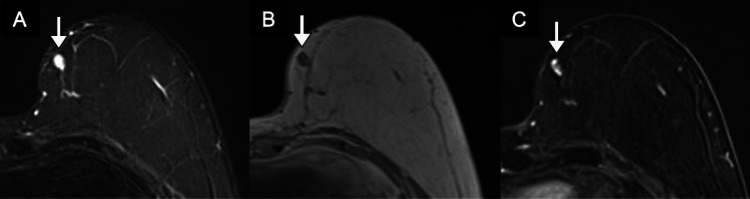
Surveillance magnetic resonance imaging of the left breast. (A) Axial STIR, (B) pre-contrast T1, and (C) post-contrast T1 fat-saturated images show an 8-mm oval, enhancing STIR hyperintense mass (arrows) located superficially at the contact zone. The mass demonstrated slow progressive enhancement (type 1 curve). A few additional small enhancing foci are seen along the breast-flap interface. STIR: short tau inversion recovery
